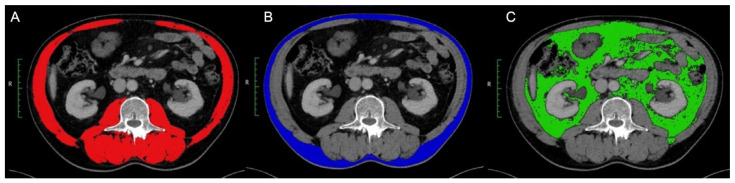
Detection of body composition within a CT scan at lumbar vertebra L3. (A) Total abdominal muscle area (TAMA) (red); (B) Subcutaneous fat tissue area (SFA) (blue). (C) Visceral fat tissue area (VFA) (green).
